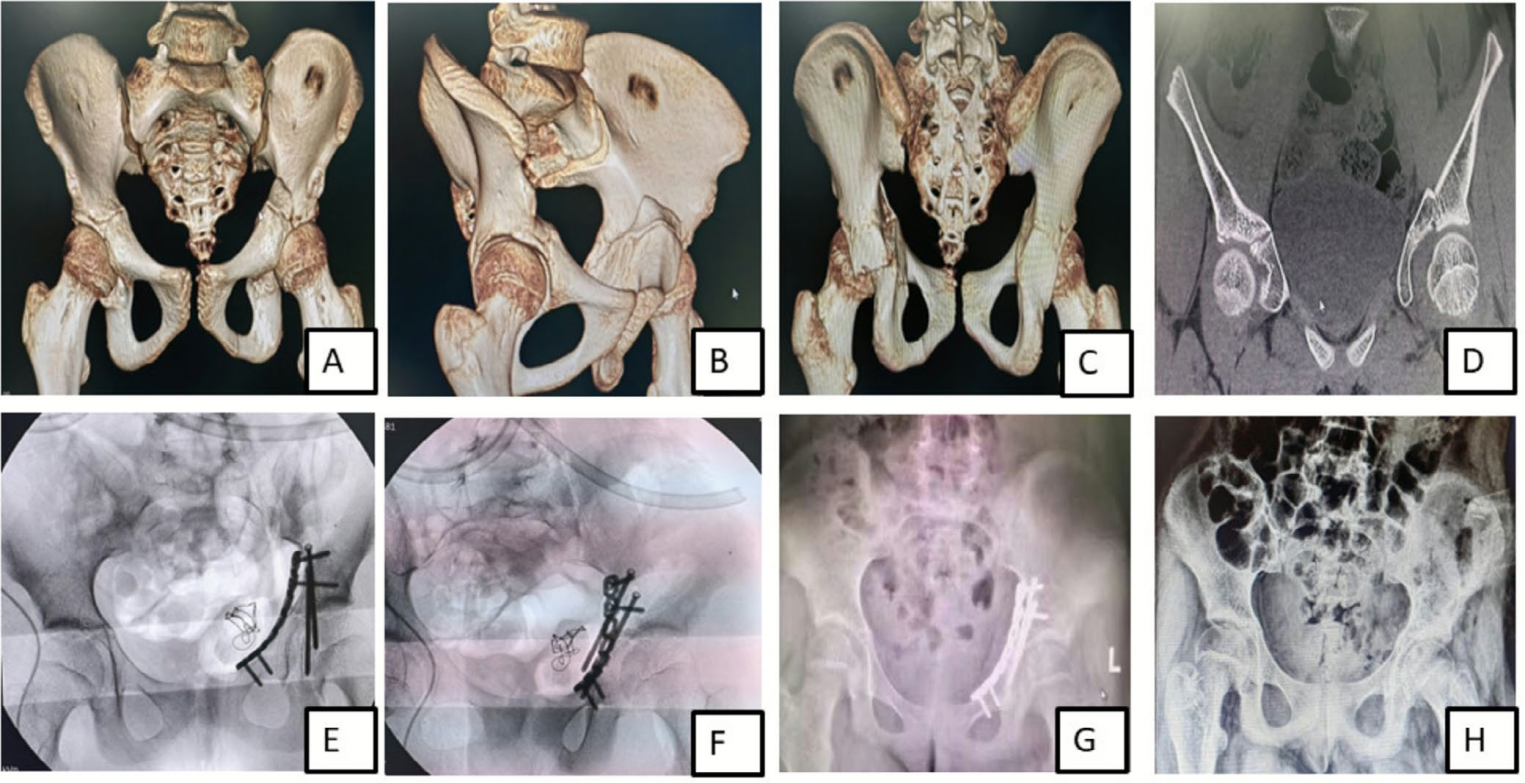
A 10‐year‐old female sustained a fall resulting in a transverse fracture of the left acetabulum and underwent surgery 5 days after the injury. CT 3D reconstruction images include the AP view (A), left oblique iliac view (B), posterior view (C), and coronal view (D), showing a transverse fracture of the left acetabulum. The posterior column was fixed with two screws, and the anterior column was reconstructed with a steel plate placed across the “Y” cartilage area (E and F). Postoperative radiograph in the AP view (G) confirmed that the fracture was reduced, fixed, and acetabular congruency restored. One year post‐surgery, the X‐ray (H) showed satisfactory fracture healing, and the internal fixation was removed.
